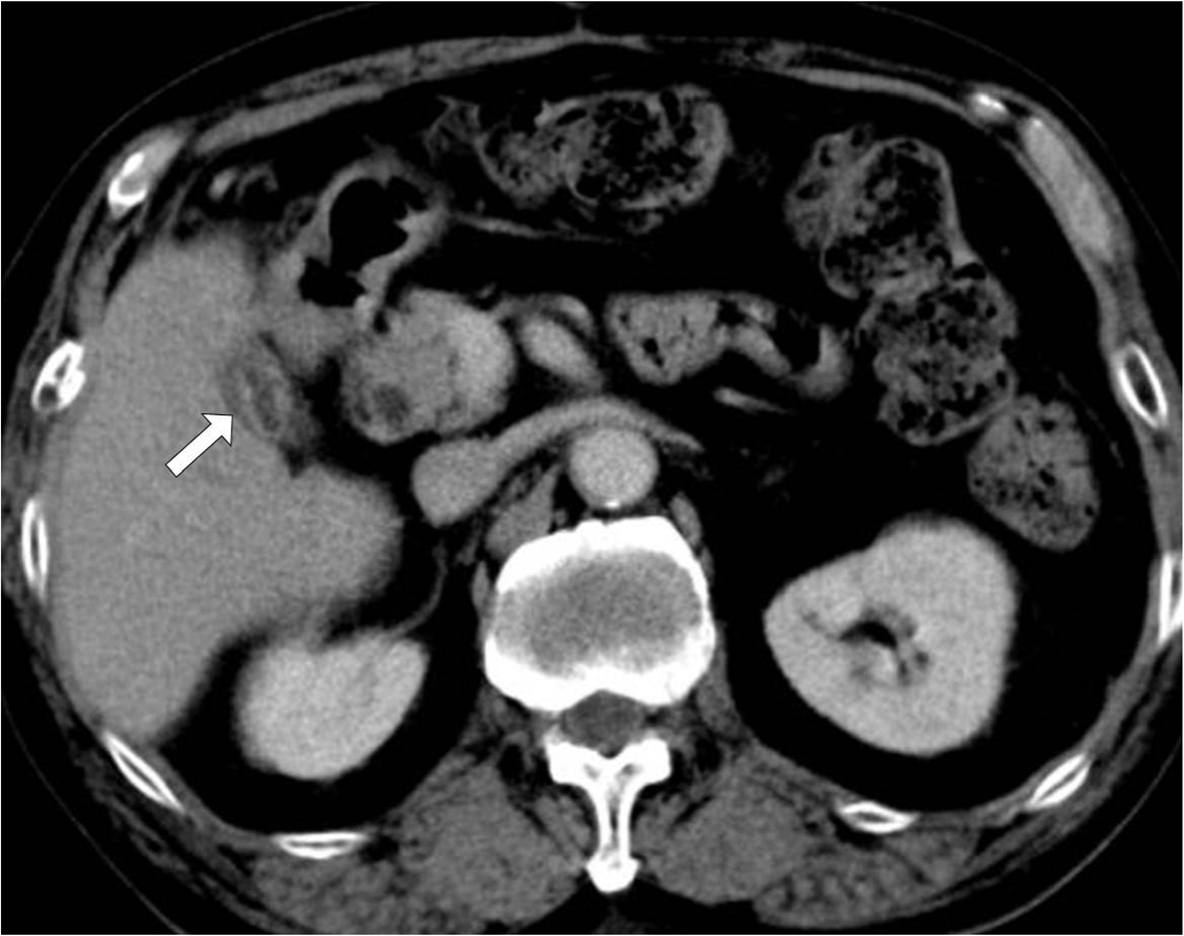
The follow-up CT scan 7 months after the surgery showed the left gallbladder was changed atrophic (white arrow)
